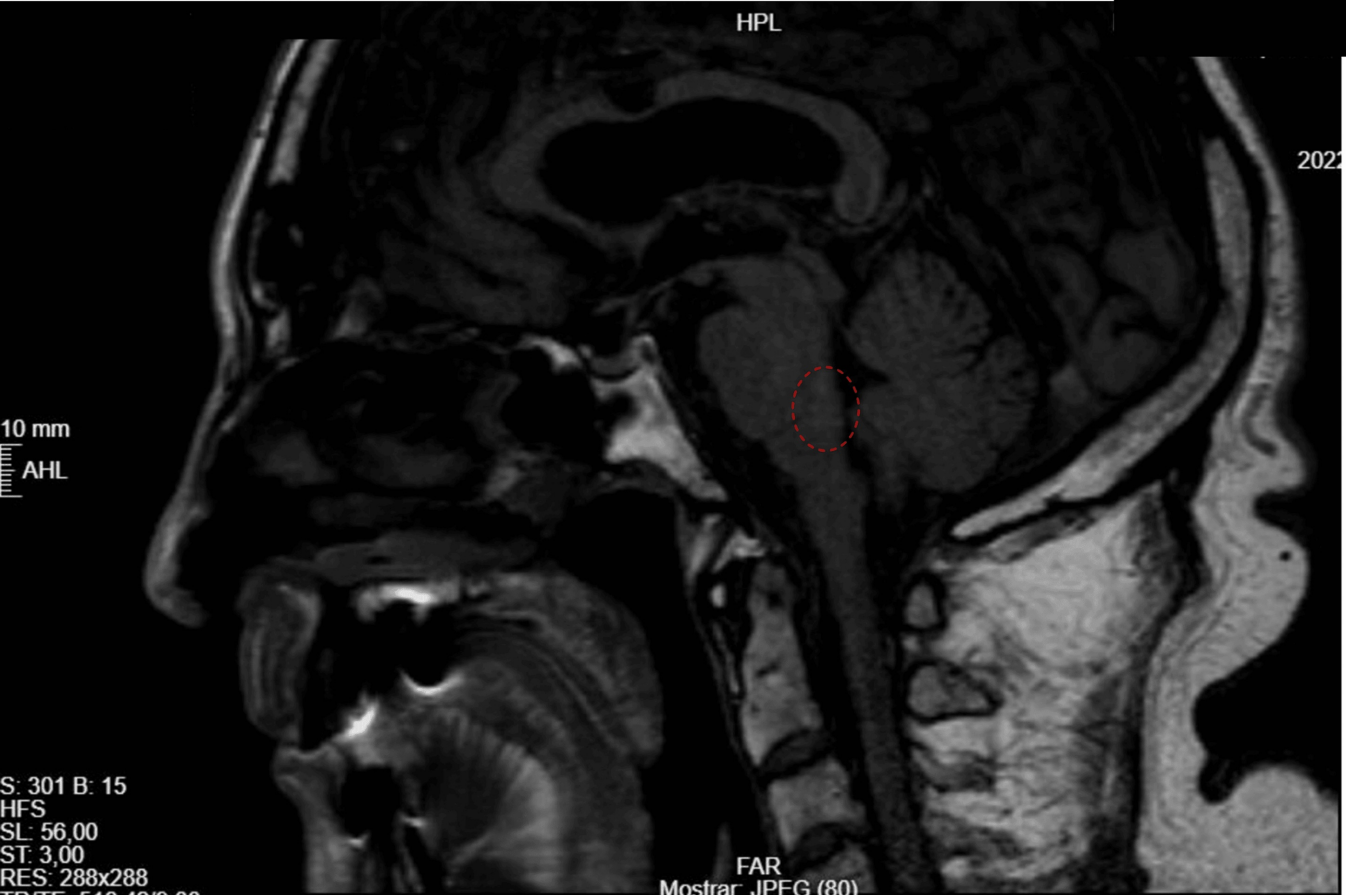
MRI confirming acute ischemic lesion in the dorsal pontine tegmentum (red circle)
